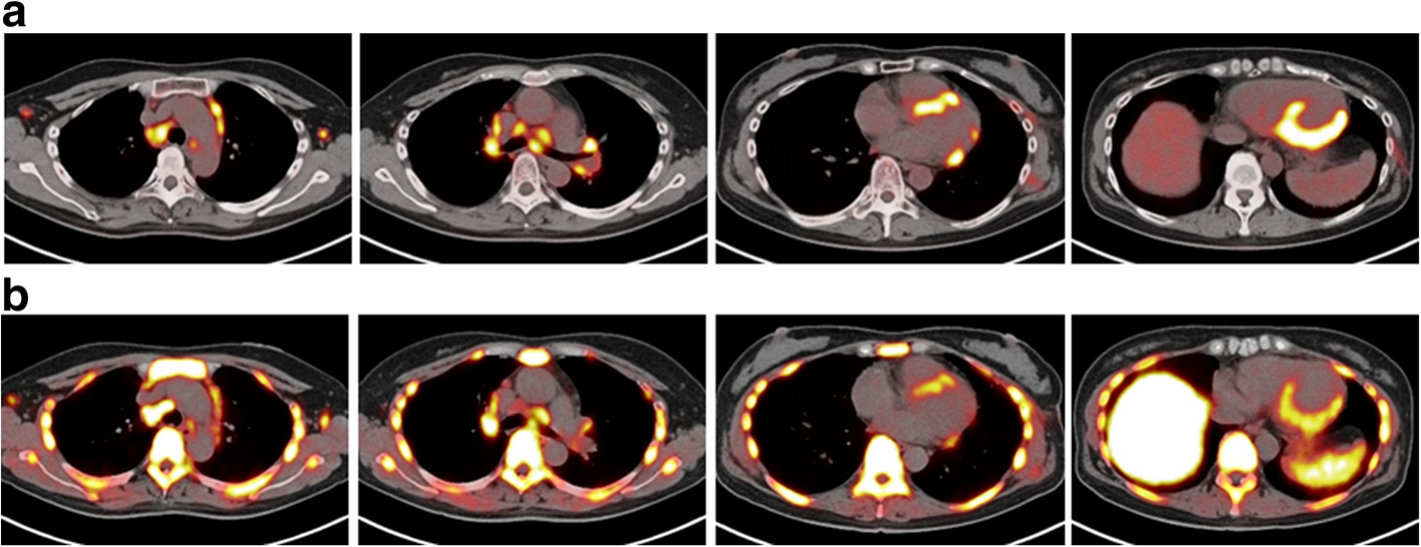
PET/CT images of a 57-year-old female with cardiac and extra-cardiac thoracic sarcoidosis (patient 1 in Table 1). Transverse ^18^F-FDG (a) and ^18^F-FLT (b) PET/CT fusion images show increased uptake in the mediastinal and hilar lymph nodes, and focal uptake at the anteroseptal, inferoseptal, and inferolateral myocardium
